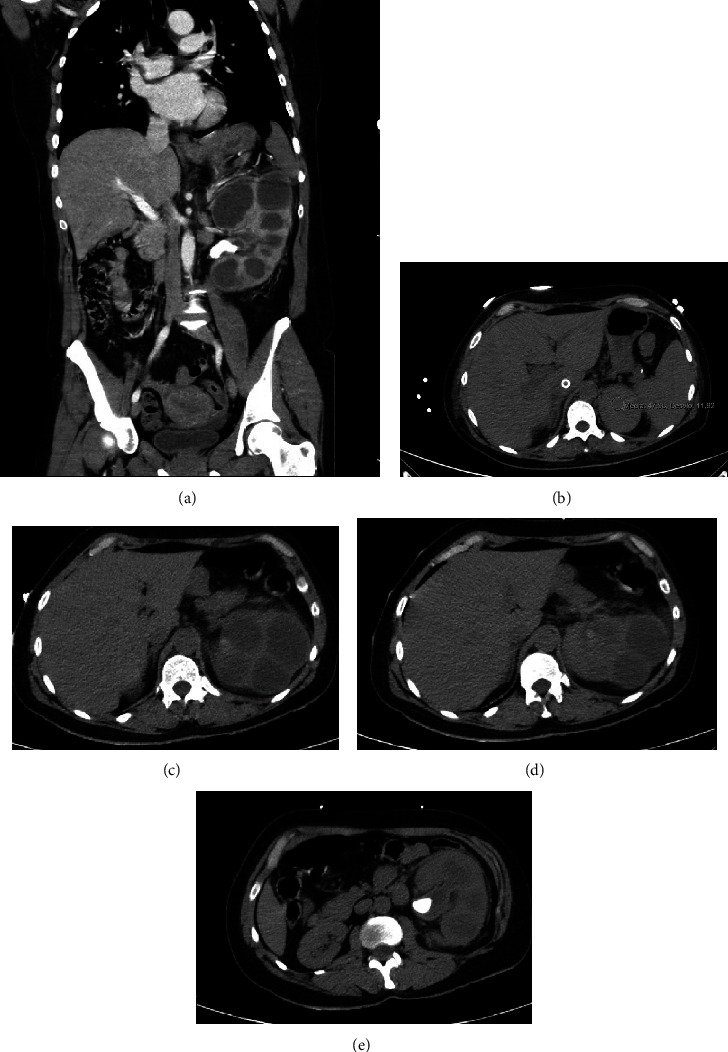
Case 1 thoracoabdominopelvic CT scan (at admission). CT scan revealed an incomplete staghorn calculus measuring 26∗14 mm impacted in the right pyelo-junction inducing hydronephrosis (a, e) and signs compatible with xanthogranulomatous pyelonephritis (a, c–e). A solid and heterogeneous right adrenal mass of 60∗55∗62 mm can also be identified (b).
